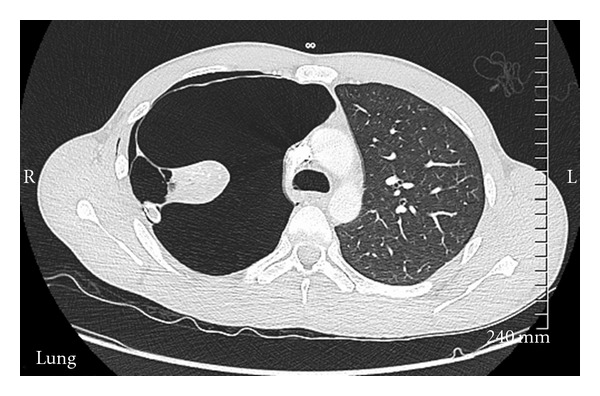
Chest CT showing bullous disease.
